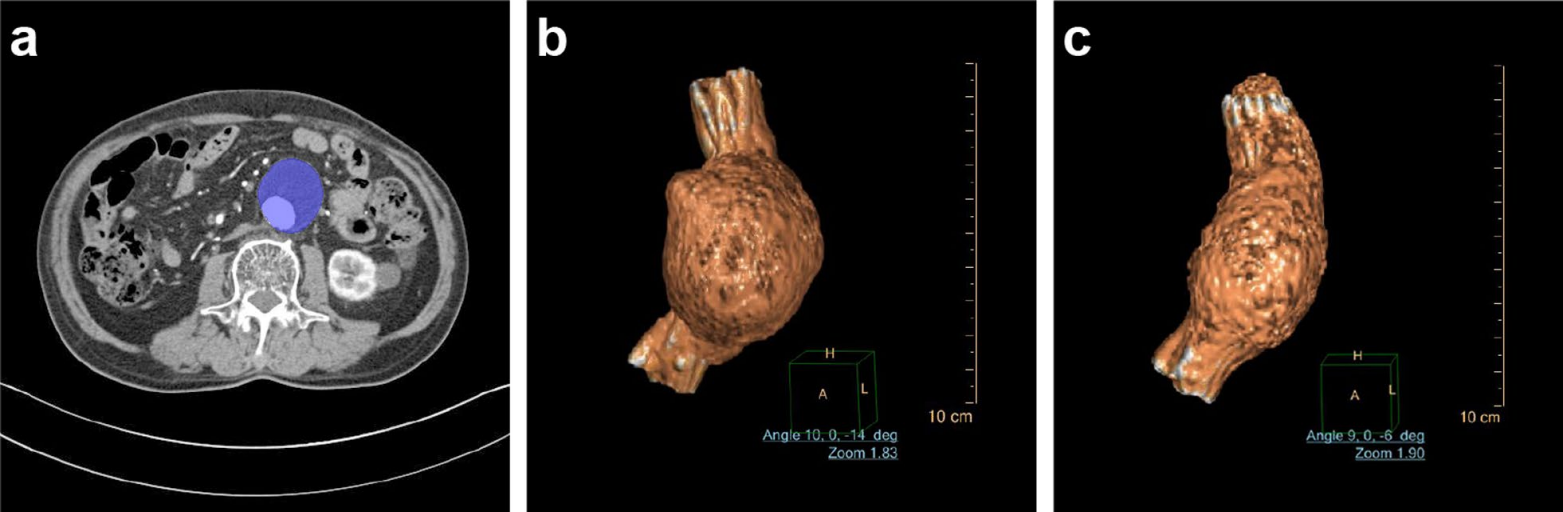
Traditional imaging evaluation. a Maximal axial plane of the abdominal aortic aneurysm (the blue area). b, c Reconstruction and volume measurement of the aneurysm based on the first and the second postoperative follow-up CT scans
